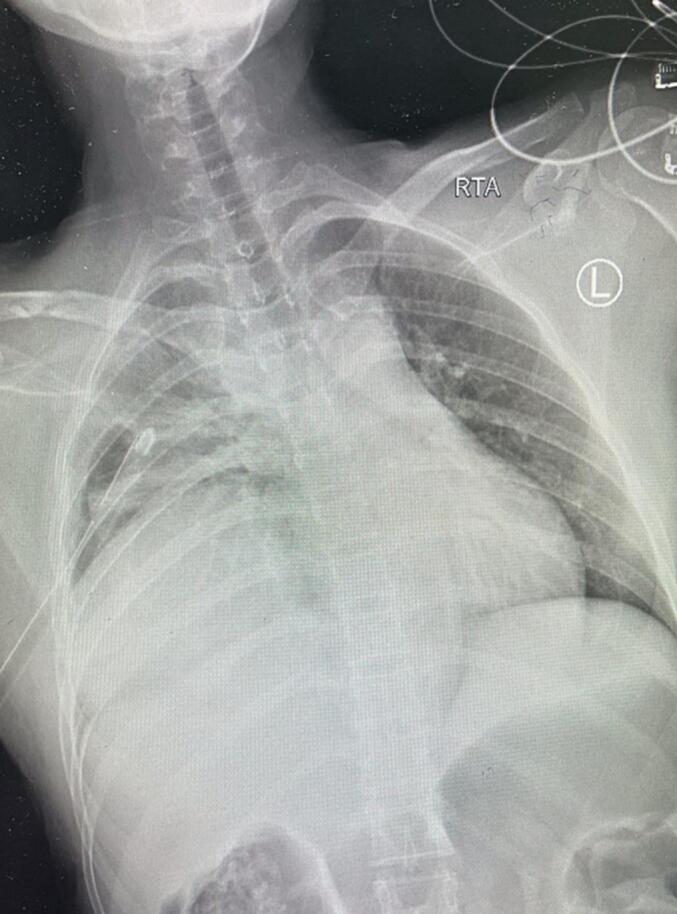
A chest x-ray indicates opacities on the right side of the chest with mid-clavicular fracture and intercostal tube in place.
